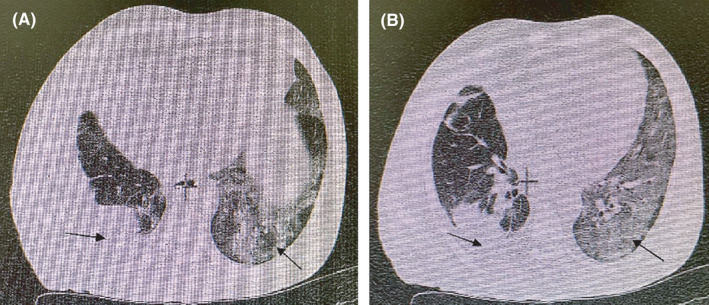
HRCT chest imaging. On the first day of hospitalization. A, Multifocal ground glass opacities in both lungs with more involvement of the left lung and large volume with a collapse of the lower lung on the right and B. multifocal ground glass opacities in both lungs with more involvement of the left lung and sporadic consolidation in the right lung
